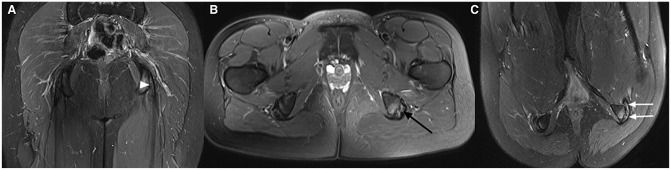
T2-weighted Dixon magnetic resonance imaging scans from a 25-year-old woman with hamstring symptoms on the left side. (A) Standard coronal image shows inhomogeneous hamstring tendons (arrowhead). (B) Standard axial image also shows some irregularity on the medial surface of the tendons (black arrow). (C) An axial image with hip flexion uncovers a quite large tear of the proximal hamstring tendons (white arrows).
